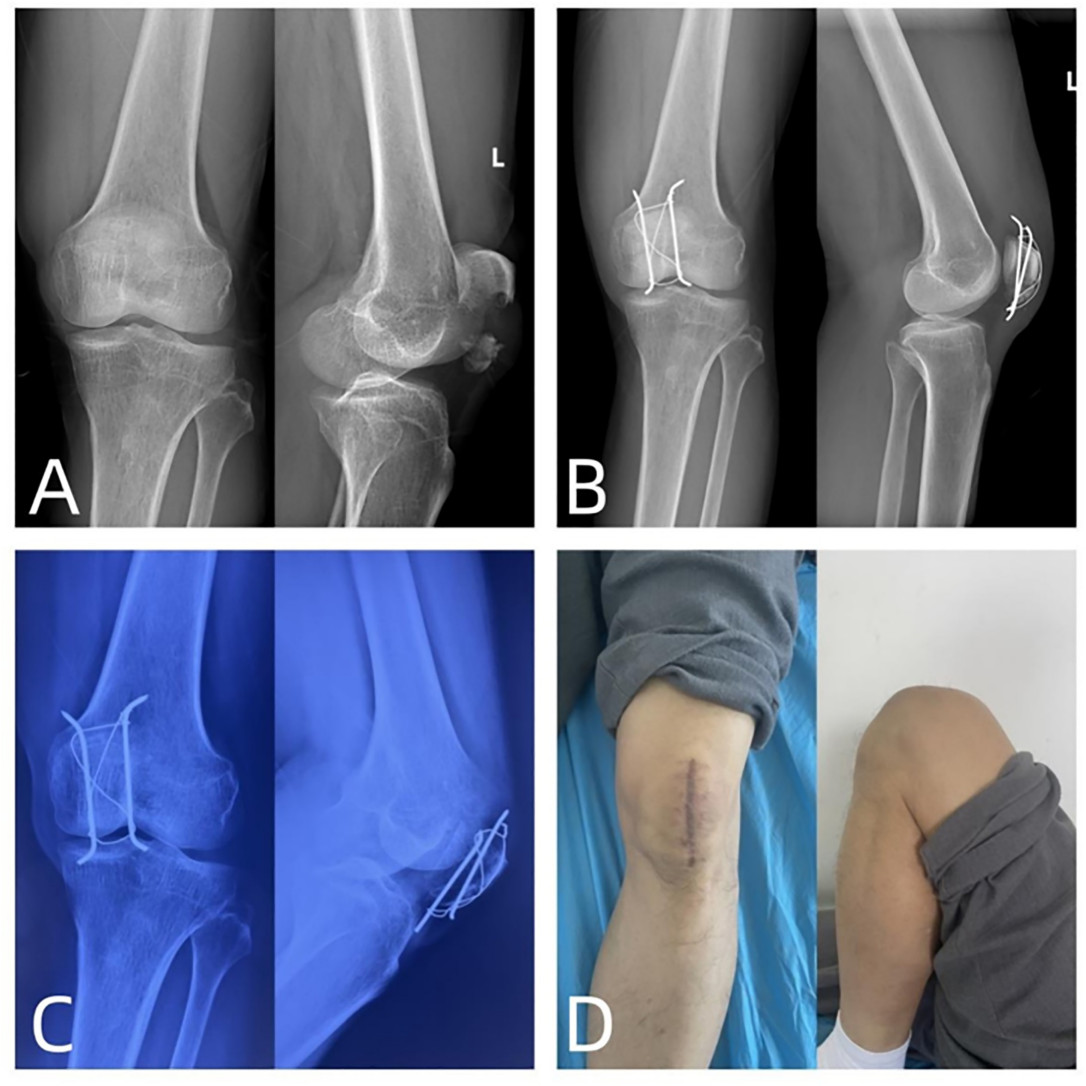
A patient is a 56-year-old male who sustained a comminuted fracture of the left patellar inferopolar region due to a fall. A: Preoperative left knee joint X-ray anteroposterior and lateral views. B: On the first day postoperative, anteroposterior and lateral X-ray images of the left knee joint were taken, and the anchor pin was not visualized on the X-ray. C: Eight months postoperative, anteroposterior and lateral X-rays of the left knee joint show that the fracture has clinically healed according to the standards. D: Functional status of the left knee joint mobility at eight months postoperatively.
